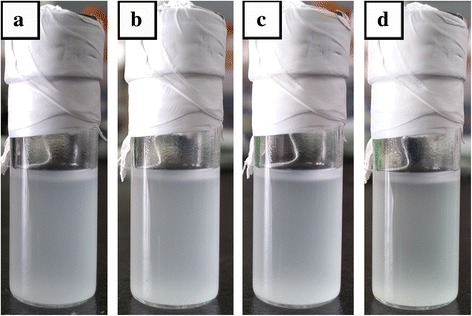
Dispersion of LiCl in toluene after ultrasonic treatment without and with different aging time: (a) without aging time, (b) 1 min, (c) 3 min, (d) 5 min
